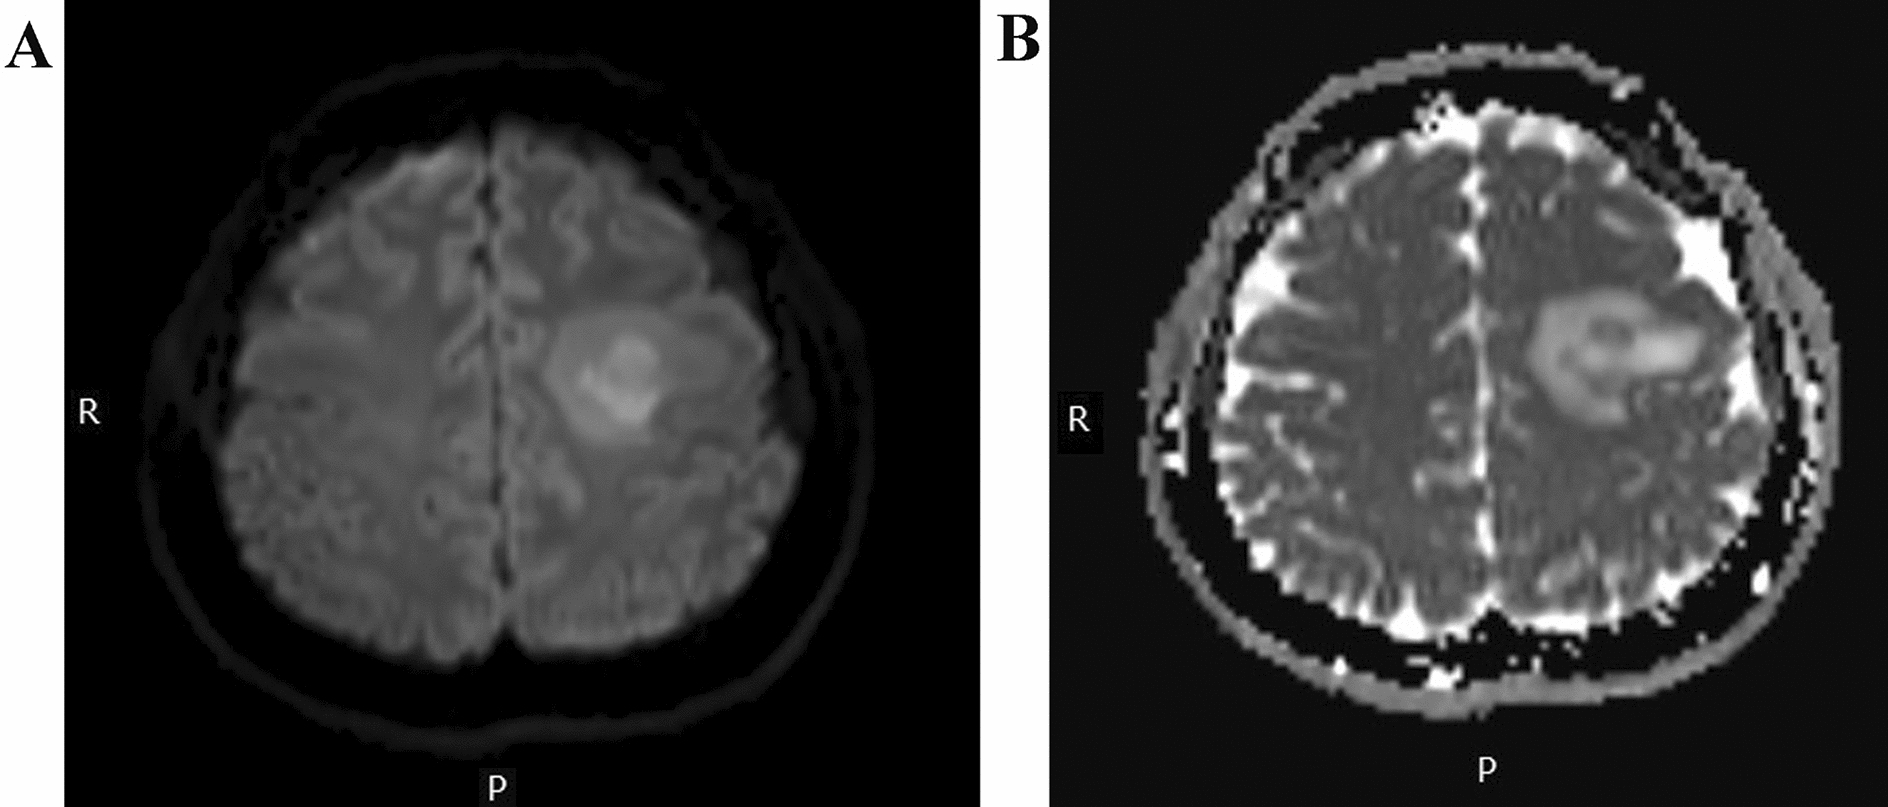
Before surgery, diffusion-weighted magnetic resonance imaging (DW-MRI) and apparent diffusion coefficient (ADC) mapping showed an inhomogeneous hyperintense area within the left centrum semiovale center. DW-MRI revealed a core of hyperintensity, which was hypointense on the ADC map. A DWI image (*b* = 1000 s/mm^2^). B ADC map
